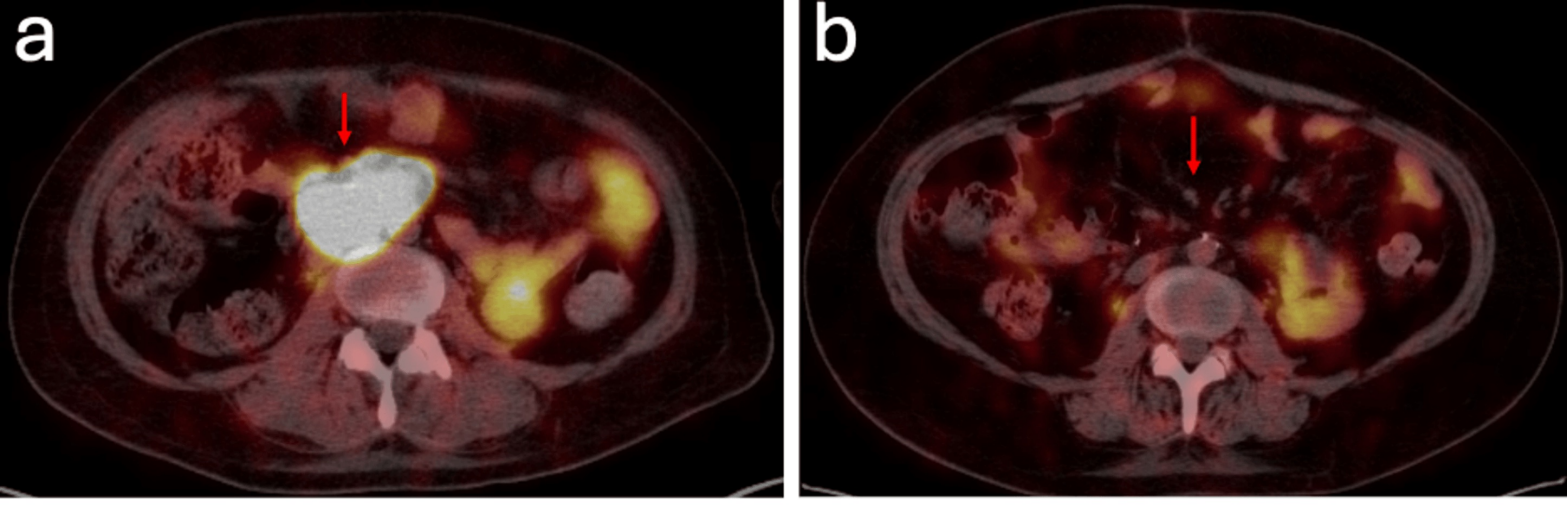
Pre and postoperative Cu-64 Dotatate PET/CT demonstrating resolution of radiotracer uptake following gross total resection of paraganglioma. (a) Axial images obtained from fused Cu-64 Dotatate PET/CT scan preoperatively demonstrate uptake of radiotracer in the tumor (red arrow). (b) Postoperative repeat imaging three months later demonstrated gross total resection of tumor without hypermetabolism to suggest residual or recurrent tumor, presumed paraganglioma. Normetanephrines were diminished postoperatively.
